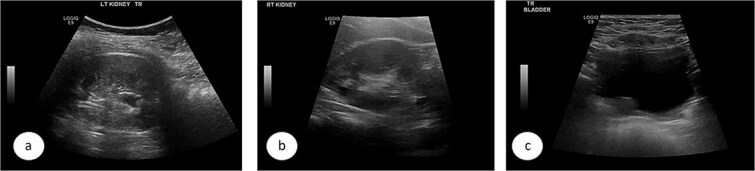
Ultrasound images showing the left (a), and right (b) kidneys and the urinary bladder (c). VCUG is showed bilateral grade 3 VUR (d). DMSA images are shown in (e).
